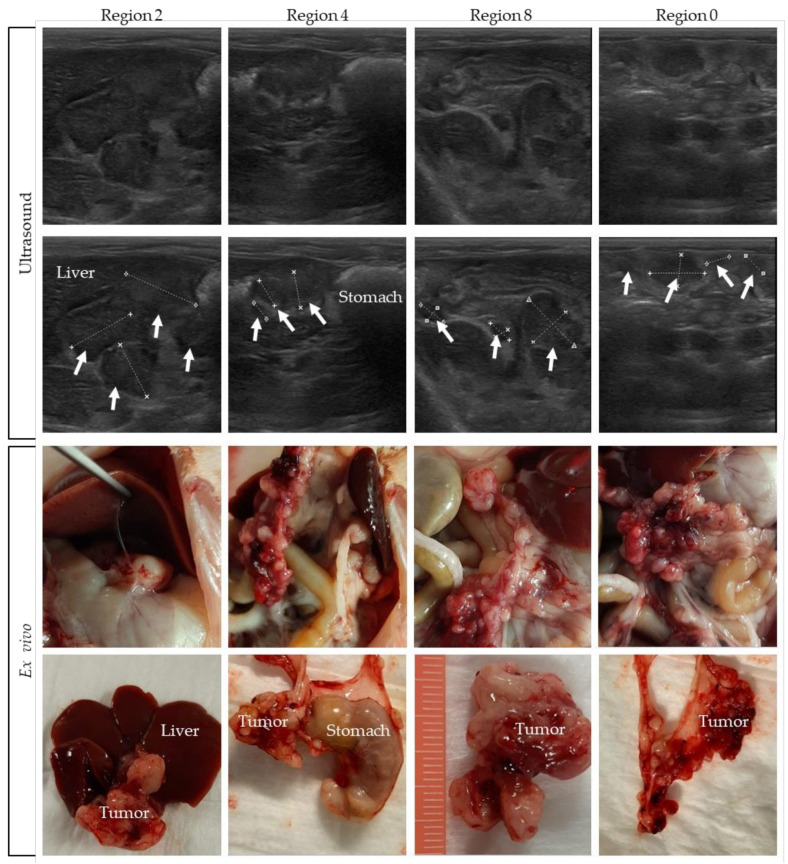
Tumors on ultrasound compared to the ex vivo tumor load. Tumor lesions are indicated with white arrows, and organs are named.
